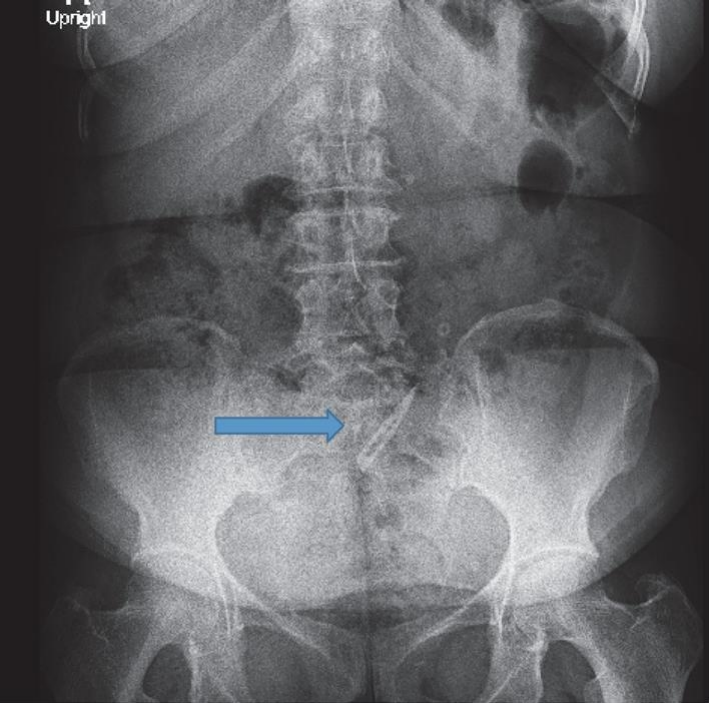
Abdominal X-ray in upright position showing surgical blade in the abdomen.
